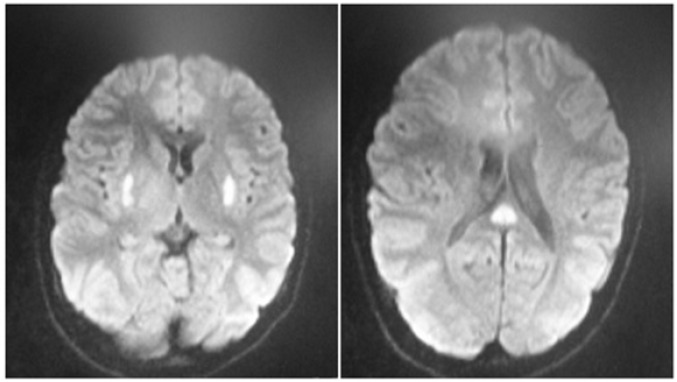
Bilateral basal ganglia hyper-intensities on brain magnetic resonance imaging (MRI
